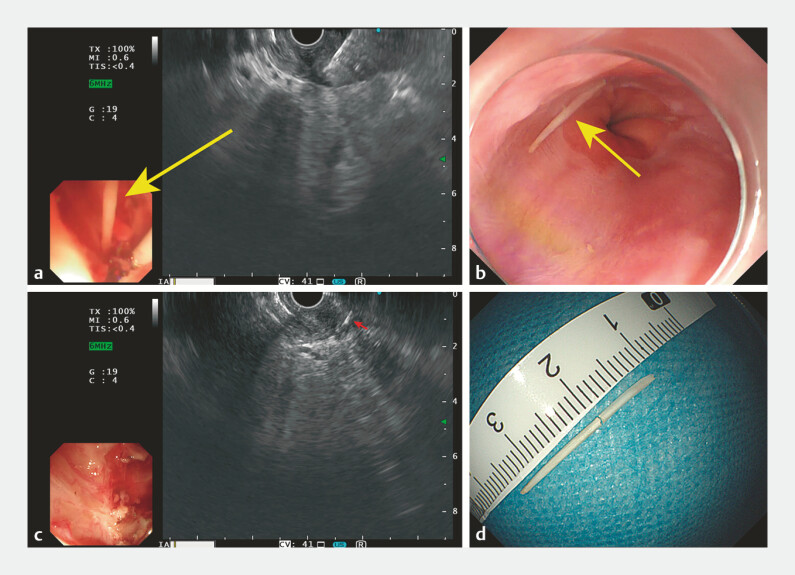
Images of endoscopic ultrasonography (EUS)-guided biopsy forceps removal of the two parts of a fish bone showing:
